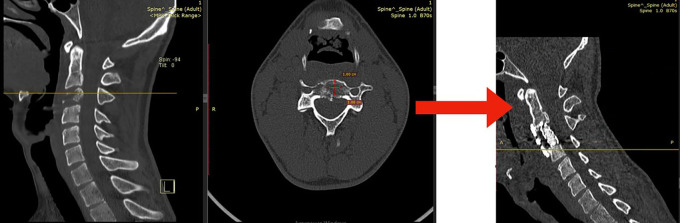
A computed tomography (CT) image taken after surgery reveals a titanium cage and plating were used in conjunction with an anterior cervical corpectomy and discectomy.
